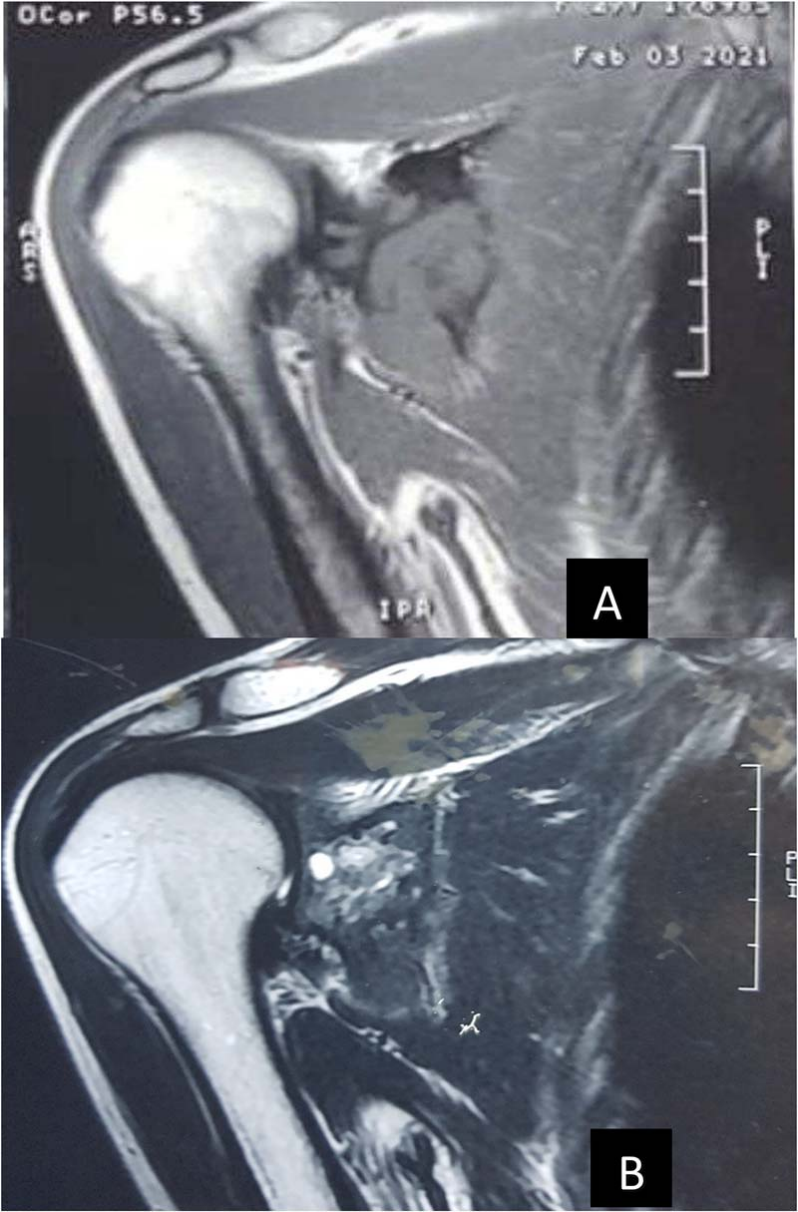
MRI images of the right shoulder displayed a high signal intensity lesion, (A) T1-weighted MRI demonstrates decreased signal at the right scapula and (B) T2-weighted MRI.
